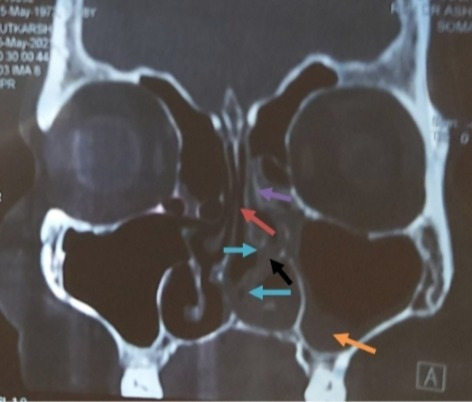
Coronal section of CT PNS showing occlusion of left maxillary ostium (black arrow), moderate mucosal thickening in the left maxillary sinus with air-fluid level (orange arrow), moderate mucosal thickening of the left ethmoidal sinus (purple arrow), nasal septum deviated to right side (red arrow), mucosal hypertrophy of bilateral inferior and middle turbinates (left more than right shown by blue arrows)
